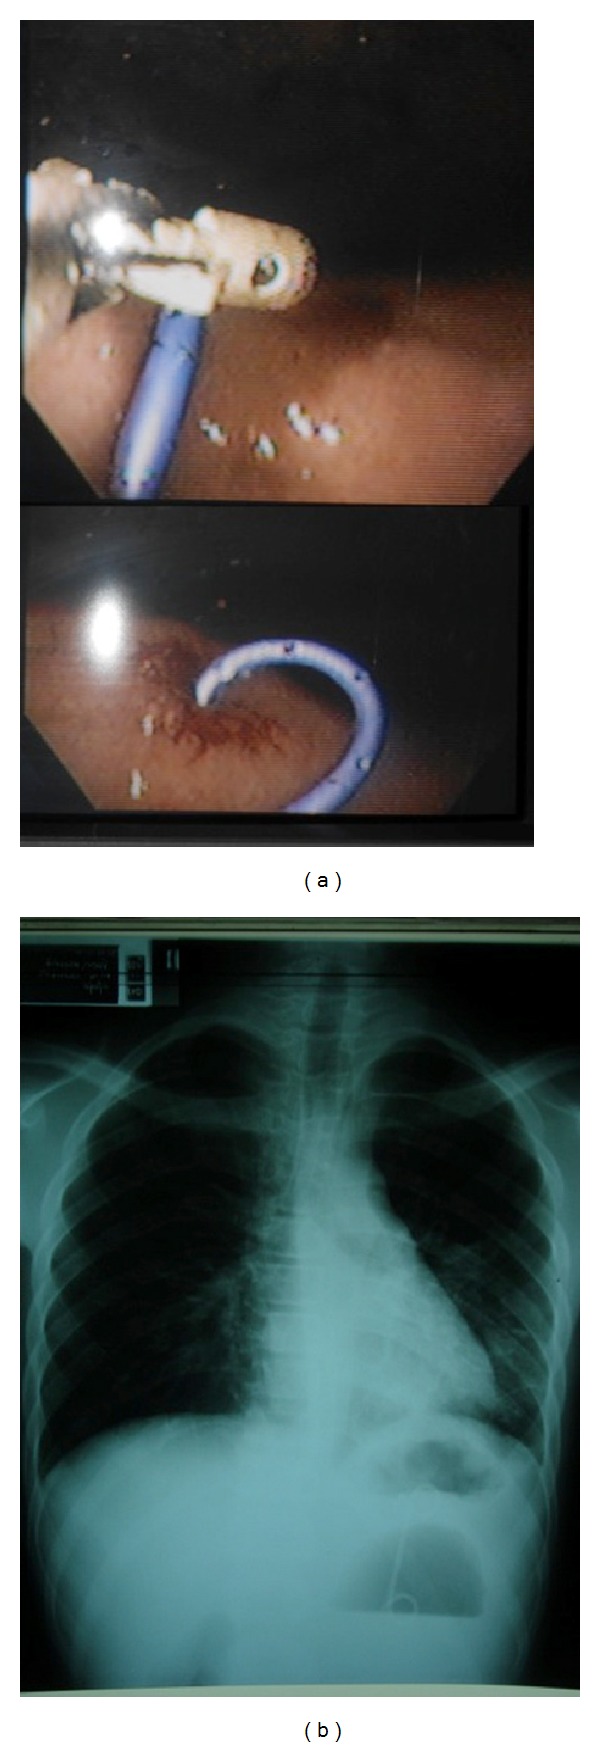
Placement of the end of the pigtail stent within the gastric lumen. Position confirmed on endoscopy (a) and on plan radiographs (b).
